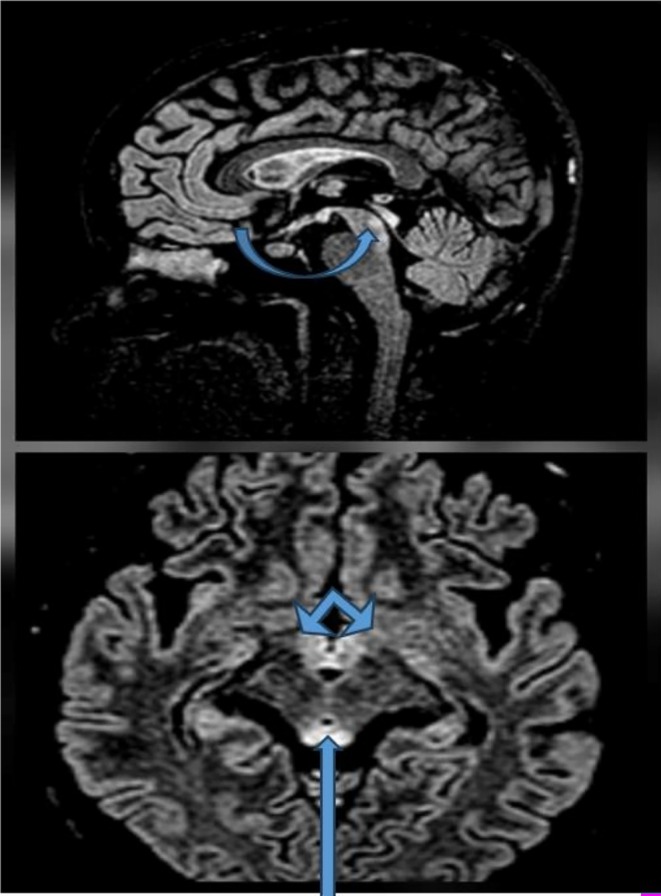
MRI of the brain (T2 sequence) showing high intensity signal in the periaqueductal gray matter and mammillary bodies.
